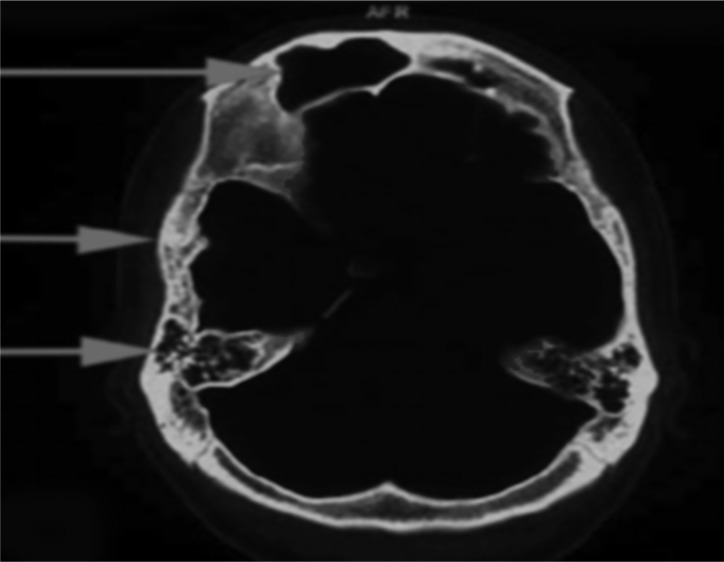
Computed tomography scan showing hyperpneumatization of frontal sinus, thickened calvarial bone with hyperpneumatisation of mastoid air cells
